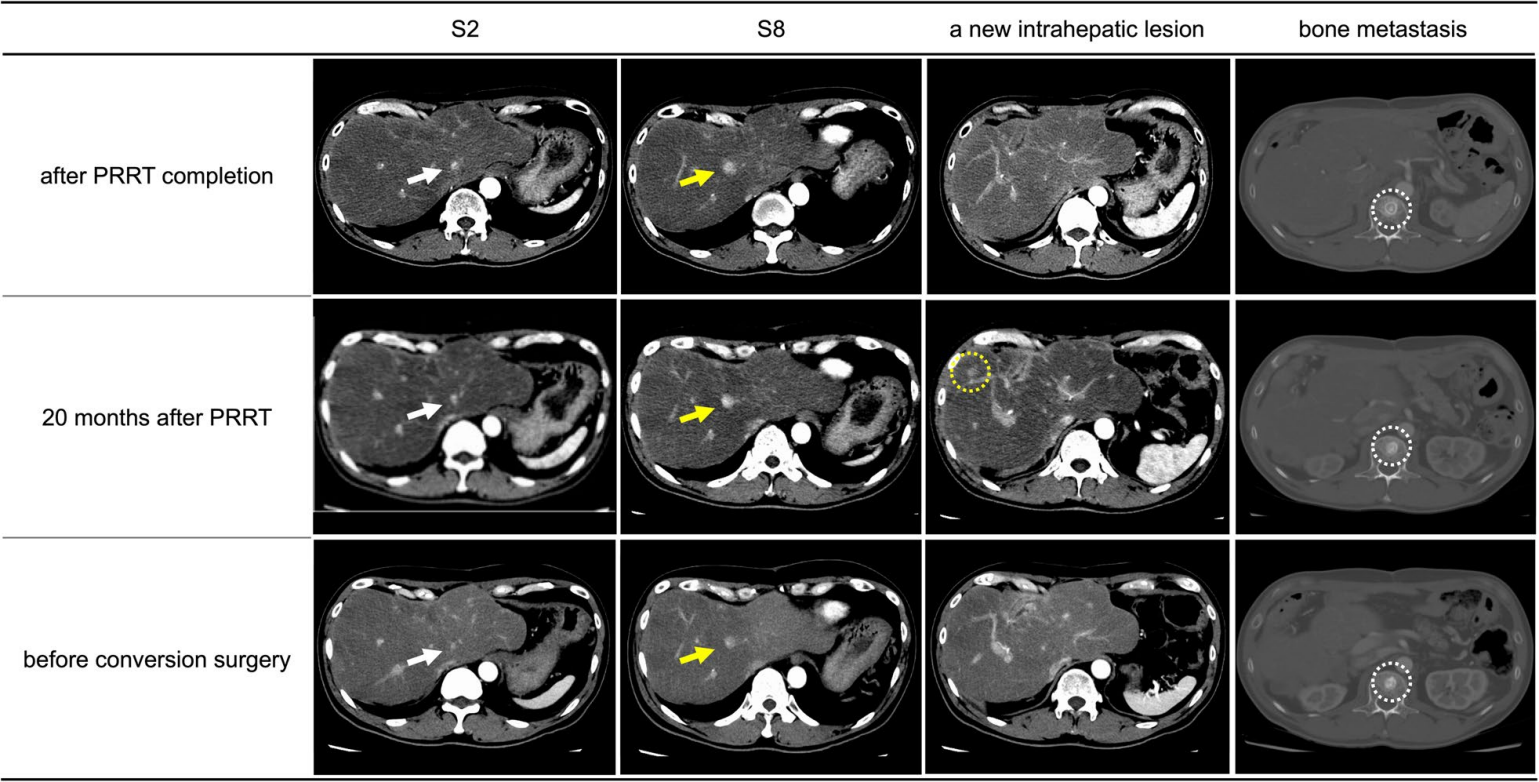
Post-PRRT to conversion surgery (contrast-enhanced computed tomography images). Most liver metastases diminished on imaging during peptide receptor radionuclide therapy, and only two reduced lesions with blurred contrast enhancement were detected in segments 2 (white arrow) and 8 (yellow arrow). A new intrahepatic lesion was identified 20 months after completion of PRRT (yellow dotted circle), however it disappeared after lanreotide administration. The bone metastasis remained stable during the treatment (white dotted circle)
